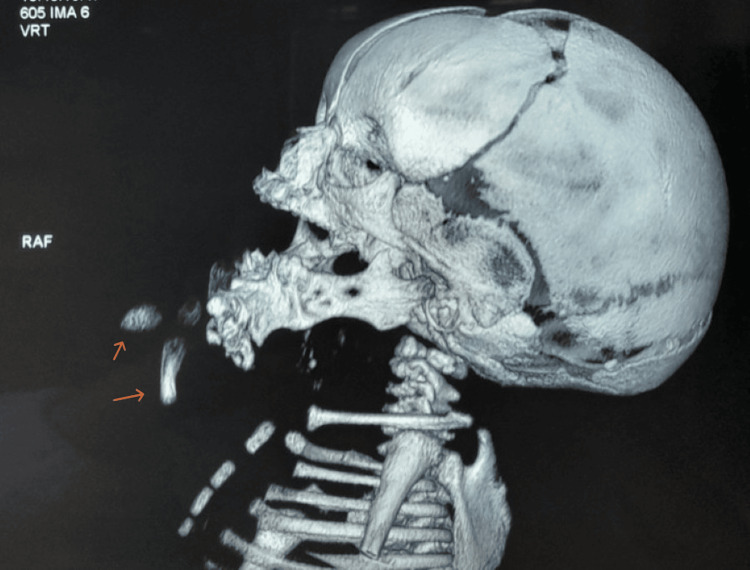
Left lateral view of a cervicofacial CT scan with 3D reconstruction showing osseous structures within a left anterolateral mentonian mass, likely corresponding to long bones of a parasitic twin (arrows), associated with mandibular malformations.
